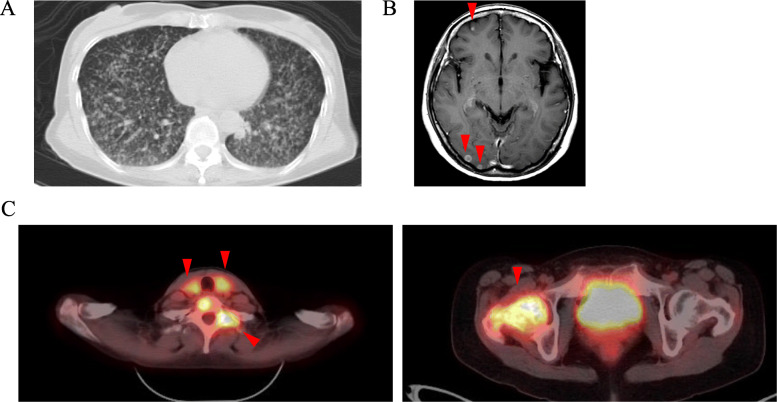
Initial CT, MRI and PET-CT. a CT scan showed diffuse pulmonary nodules in both lungs. b MRI showed multiple nodules in the cerebral hemispheres. (arrowhead) (c) PET-CT demonstrated high FDG uptake in the thyroid, subclavicular lymph nodes, and multiple bones. (arrowhead)
